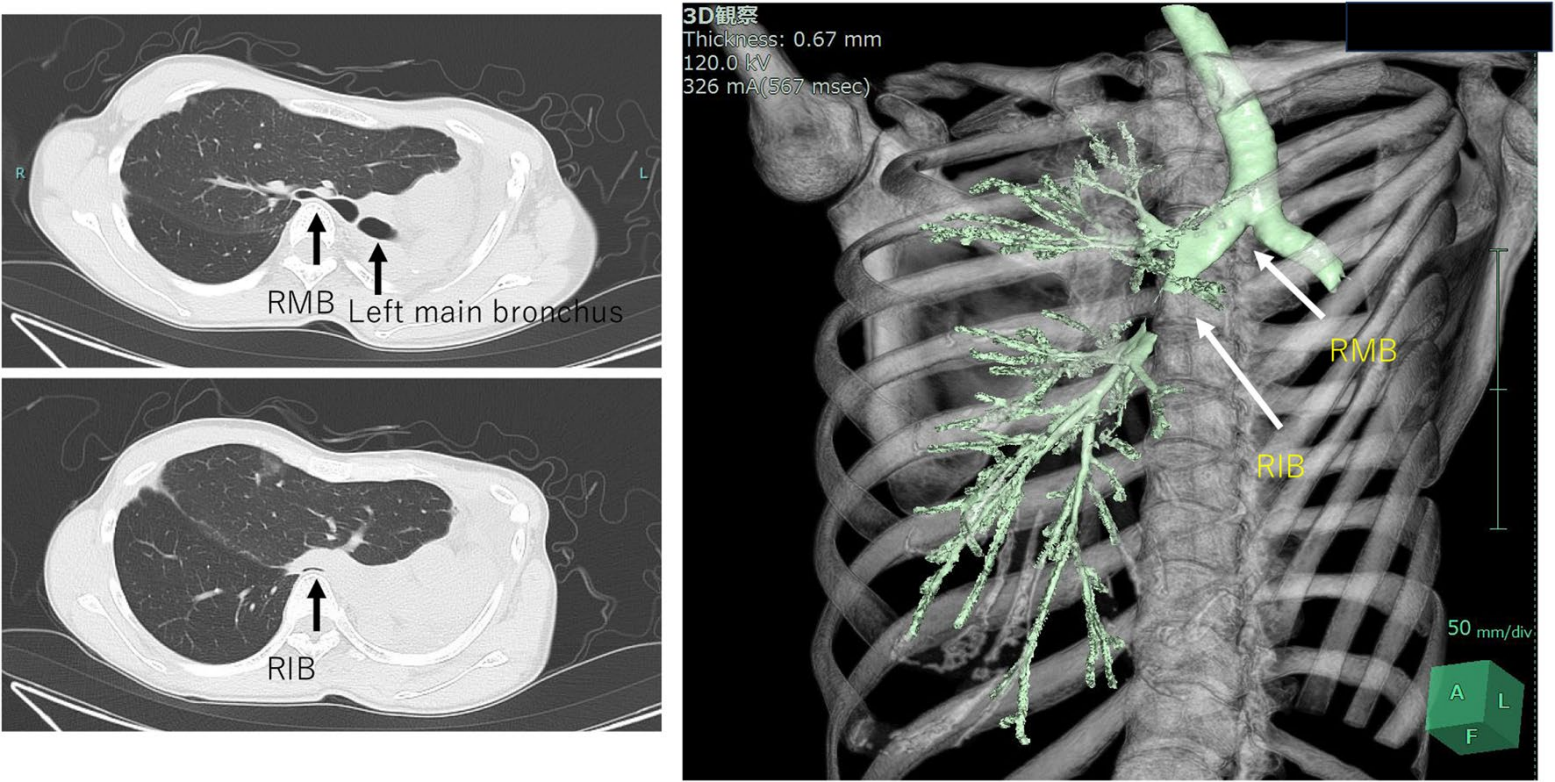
CT scan showed that the right main bronchus (RMB) and right intermediate bronchus (RIB) were compressed by adjacent vertebral bodies, which resulted in constriction. The 3D-CT image provides an overview
